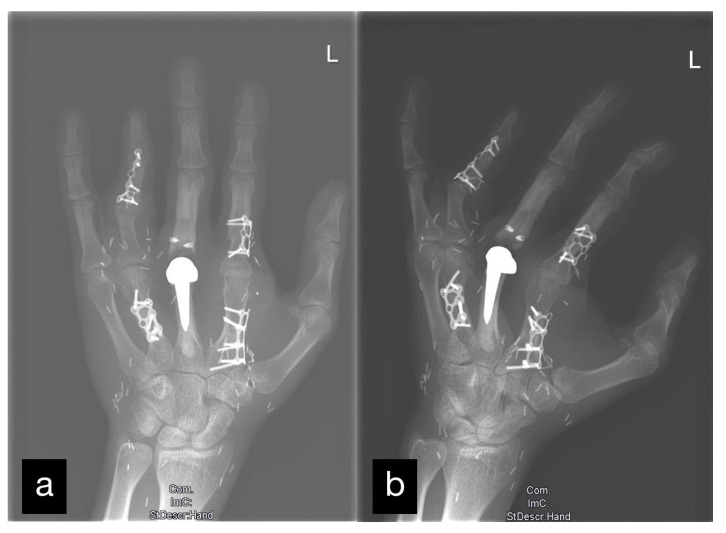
X-ray of the left hand after all mentioned reconstructions. Two levels: (a) a.p. and (b) oblique.
